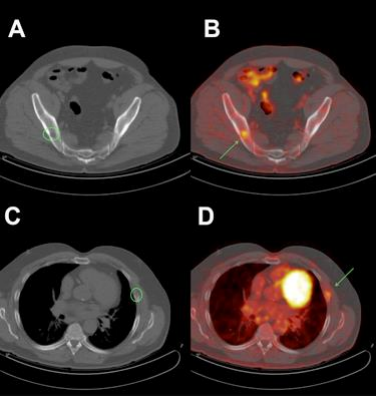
Panel of ^18^F-FDG PET-CT imaging at 6 months follow-up of patient 7. There are 2 FDG-avid bone localizations strongly suspected of being metastases (A, B). The low-dose CT scan without contrast shows cystic lesions in the right iliac bone (A, green circle) and in the fifth left costal rib (C, green circle). Both cystic locations correlated with the “hot spot” FDG uptake as shown in B and D (green arrows).
